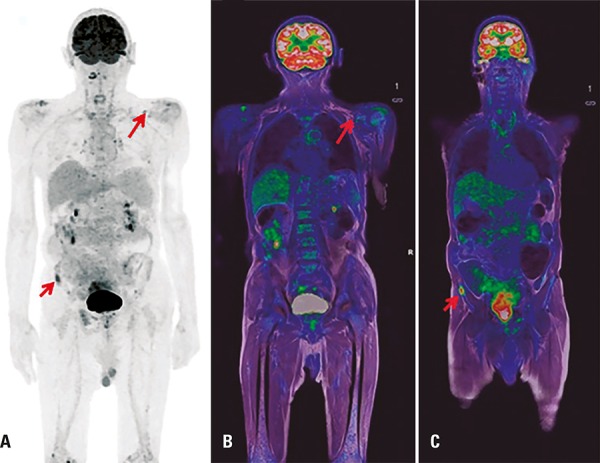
Post-chemotherapy whole-body 18F-FDG PET/MRI images of a male patient with multiple myeloma, showing residual metabolic activity in the right iliac crest and hypermetabolic left supraclavicular lymph node (arrows). (A) PET, maximum intensity projection image; (B and C) fused PET/MRI image, coronal T1
